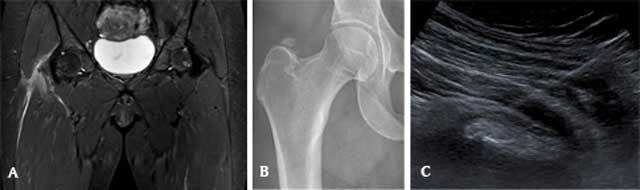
A 47-year-old woman with calcific tendinits of the right gluteus medius tendon. (A) Coronal Short Tau Inversion Recovery MRI image reveals extensive soft tissue edema (arrow) near the greater trochanter, centered around a hypo-intense calcification in the gluteus medius tendon, (B) plain radiography of the right hip confirms the calcification (arrow) in the soft tissue superior to the greater trochanter, (C) ultrasound image demonstrating the needle approaching the calcification.
